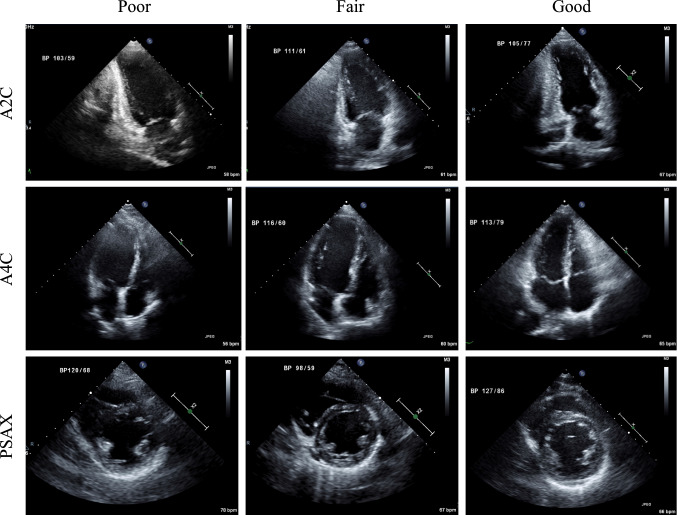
Representative echocardiography image from good to bad image quality
